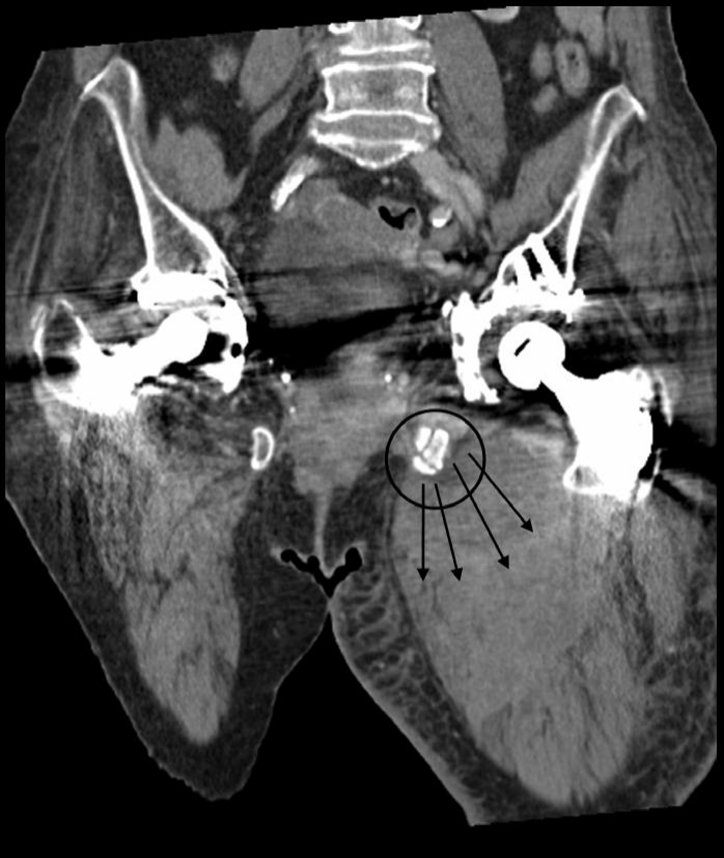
A & B: CT-scans show in the coronar view the fractures of the left anterior pelvic ring as origin of the bleeding liable for the development of the cpompartment syndrome of the left thigh.
